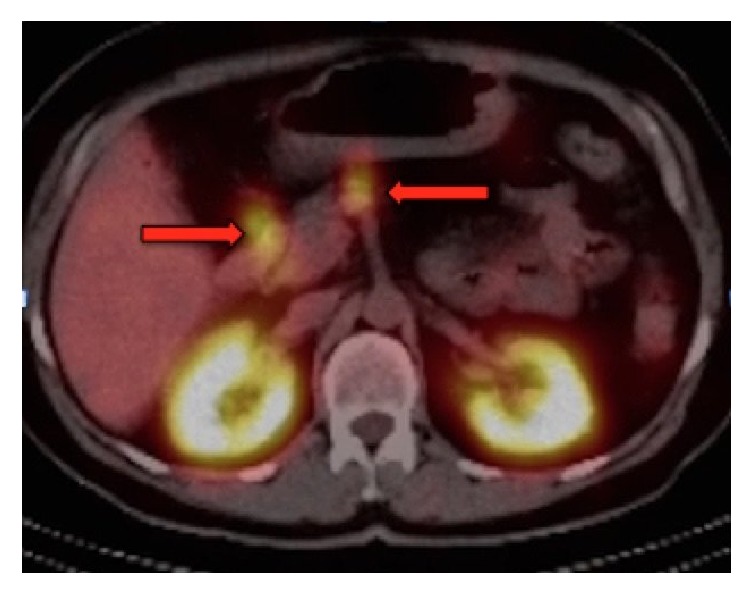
Somatostatin receptor scintigraphy (SRS) showing two small foci of moderately intense abnormal radiotracer uptake within the gastrinoma triangle.
